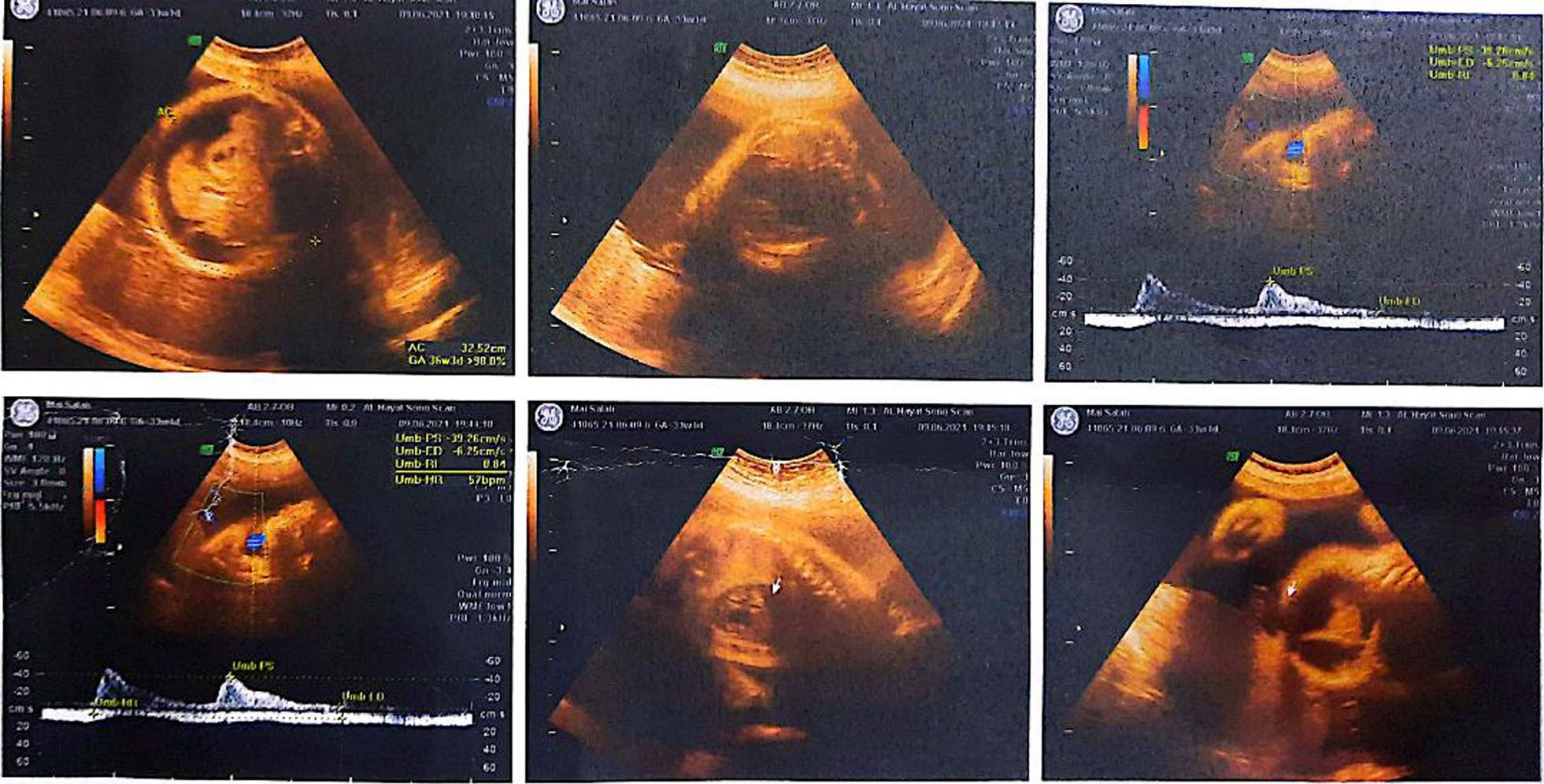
4D obstetric ultrasonography in the 34th gestational week
